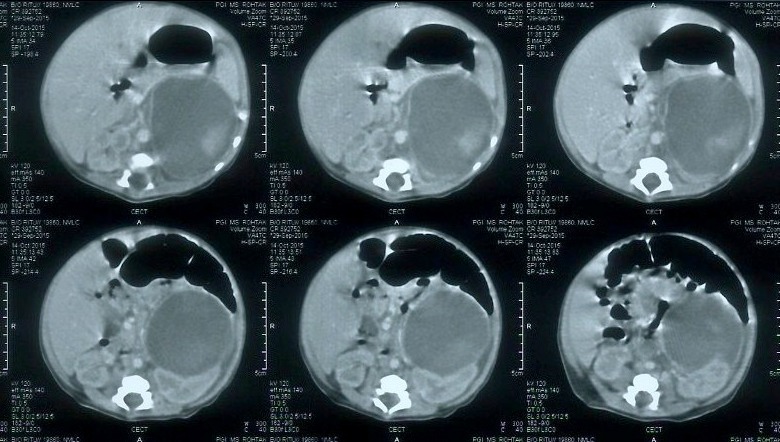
Figure 1: Computed Tomography abdomen of neonate showing large adrenal hemorrhage on left side.
